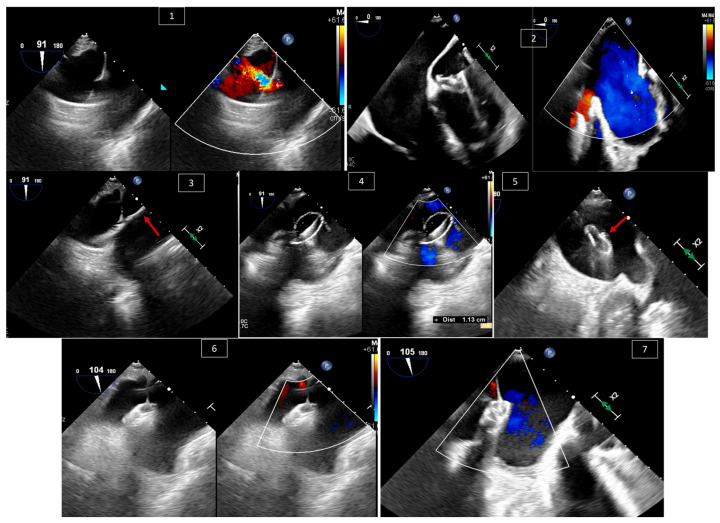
Baffle leak occlusion in a 46-year-old male patient with D-transposition of the great arteries post Mustard atrial switch operation and with a significant inferior systemic baffle leak. (1) Mid esophageal view at 90 degrees showing the systemic baffle leak with and without color Doppler, with flow crossing from the pulmonary venous baffle towards the IVC baffle. (2) Mid esophageal view at 0 degrees showing widely patent pulmonary venous baffle—easily recognized from the tricuspid valve (systemic AV-valve) with and without color Doppler. (3) Showing wire (arrow) across the systemic baffle leak. (4) Balloon sizing (1.13 cm) and occlusion of the defect with and without color Doppler. (5) Deploying the pulmonary venous baffle disc (arrow). (6) Deployment of the systemic venous baffle disc. (7) Final result with the device in a stable position and no more leaks visible by color Doppler.
